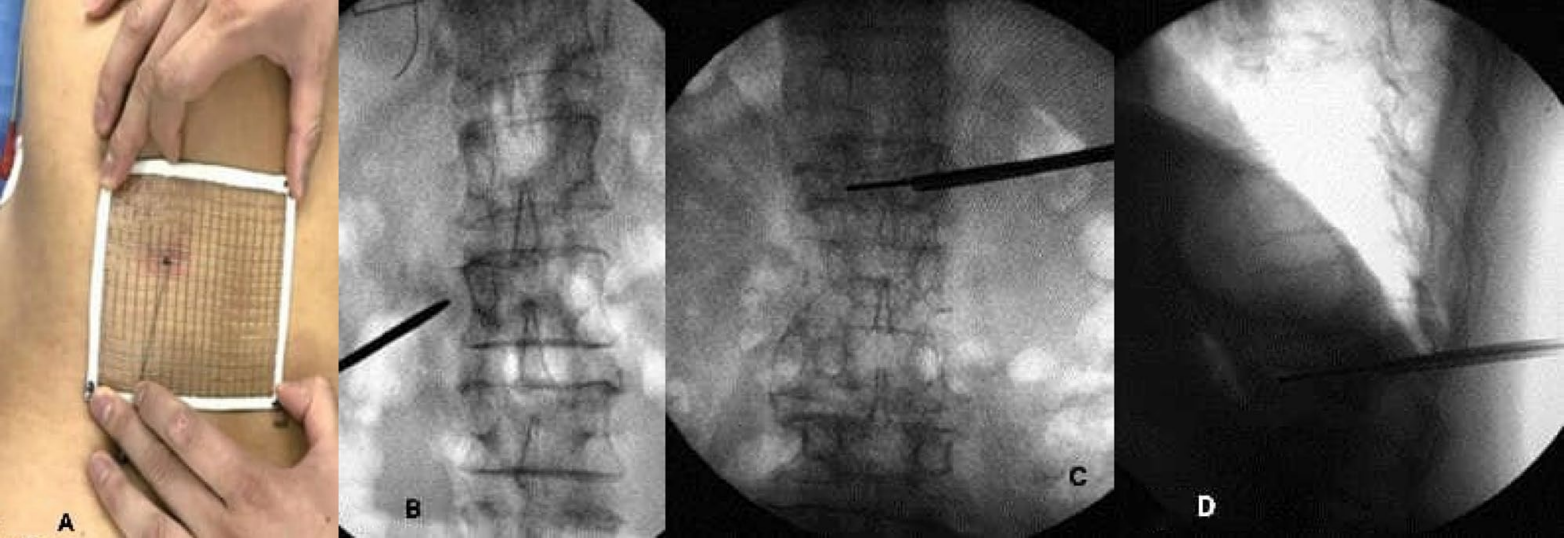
(A) Key puncture points of preoperative mesh optimization. (B) X-ray fluoroscopy showing the intersection of the midpoint of the line between the upper and lower endplates of the vertebral body and the tangent of the lateral edge of the pedicle of the vertebral body. (C) X-ray fluoroscopy to determine the target point in the vertebral body, where the tip of the guidewire reached the inner edge of the contralateral pedicle. (D) X-ray fluoroscopy showing the tip of the guidewire reaching the anterior edge of the vertebral body.
